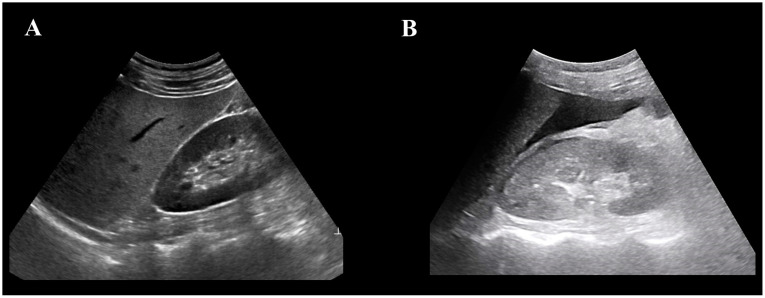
Ultrasonographic image of Morrison’s pouch. (A) Normal ultrasonographic image of Morrison’s pouch. Morrison’s pouch is observed as hyperechoic lines. (B) Ultrasonographic image with hemoperitoneum in Morrison’s pouch. Hypoechoic fluid collection in Morrison’s pouch can be observed.
